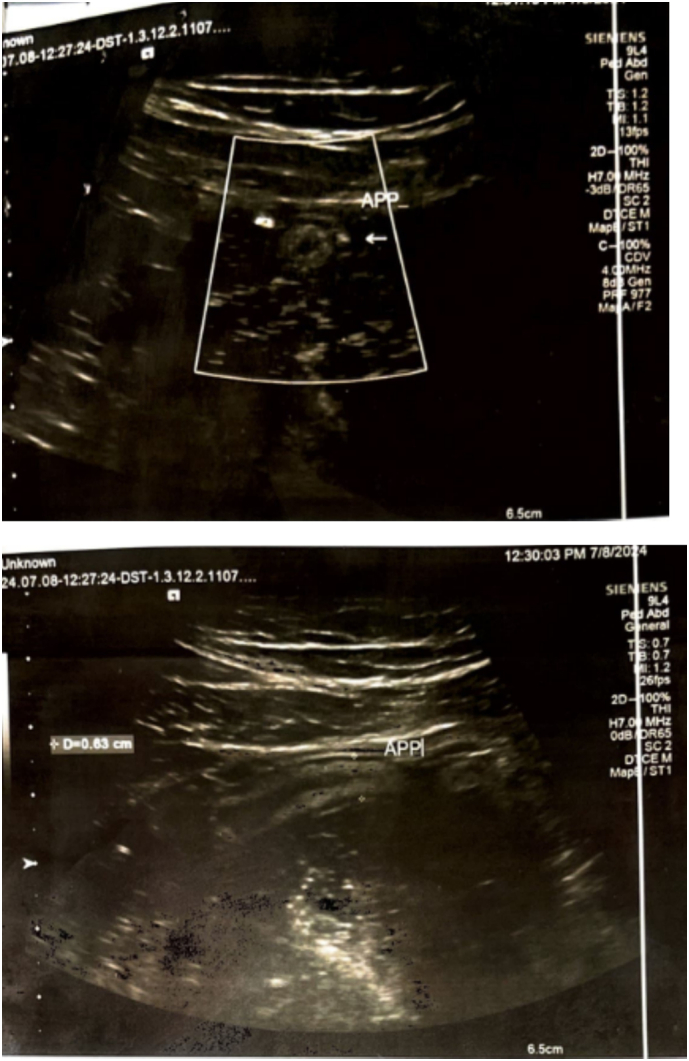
Abdominal ultrasound shows appendiceal wall thickening and increased wall to wall diameter.
